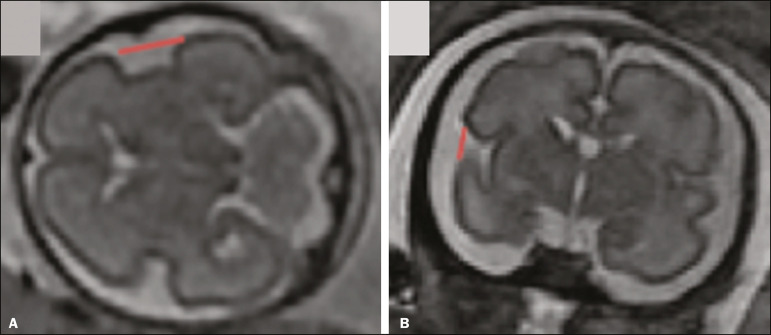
MRI measurements of anteroposterior IOD (A) and craniocaudal IOD (B).
